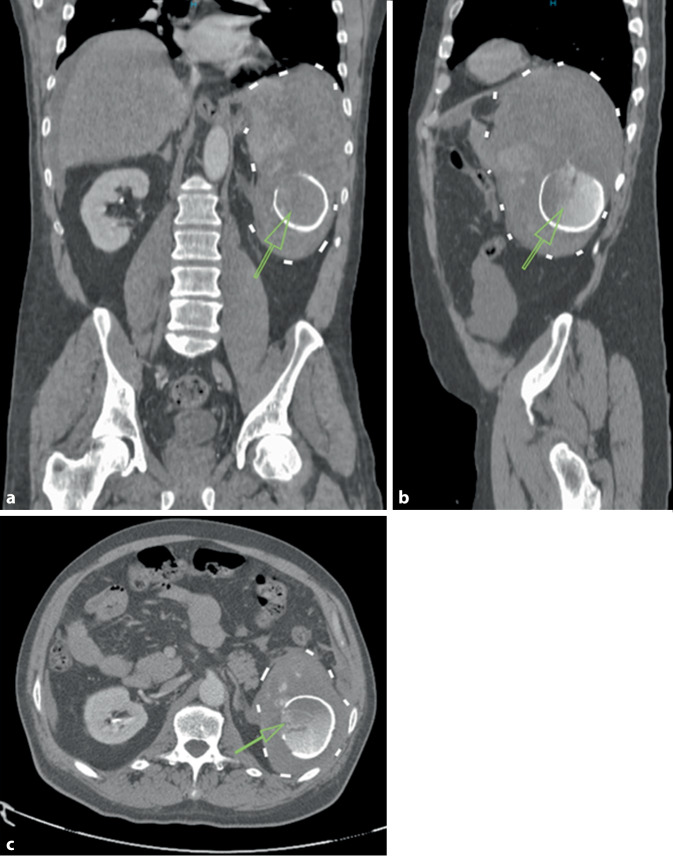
a–c Kontrastmittel-CT des Abdomens: (a) Koronare CT-Rekonstruktion mit hochgradiger Milzlazeration und perisplenischem Hämatom (*weiß*-*gestrichelt*). (b) Sagittale Darstellung mit rupturierter kalzifizierter Zyste (*grüner Pfeil*). (c) Axiale Schnittdarstellung der Zyste. Das Volumen beträgt 265 ml, Typ-IV-Verletzung der Milz nach American Association for the Surgery of Trauma (*AAST*) – splenic injury scale
